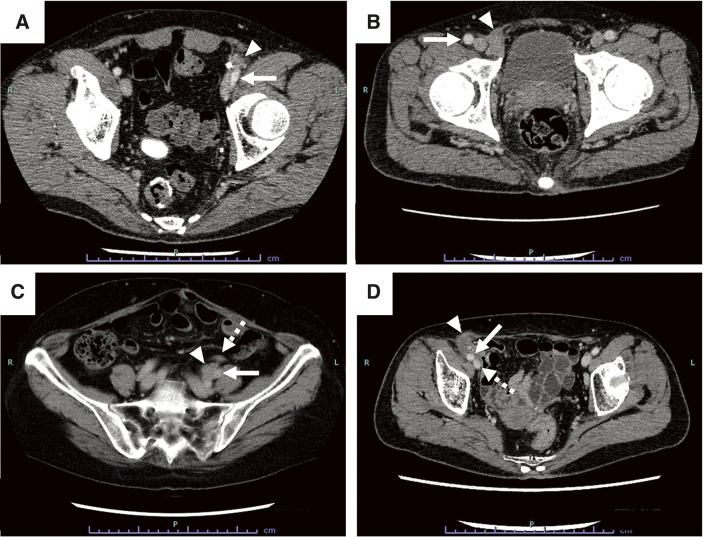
CT images showing a recurrent tumor with suspected vascular invasion in 4 cases. Arrowhead indicates the recurrent tumor in each case. (A) Case 1: An irregular nodule in contact with the left external iliac artery (arrow) was observed. (B) Case 2: An irregular nodule was observed in close proximity to the right femoral artery (arrow). (C) Case 3: A nodule was found near the left ureter (indicated by a dotted arrow), and in close proximity to the left internal iliac artery (arrow). (D) Case 4: A soft tissue nodule with calcification was located anterior to the right external iliac artery (arrow) and vein (dotted arrow). CT, computed tomography
